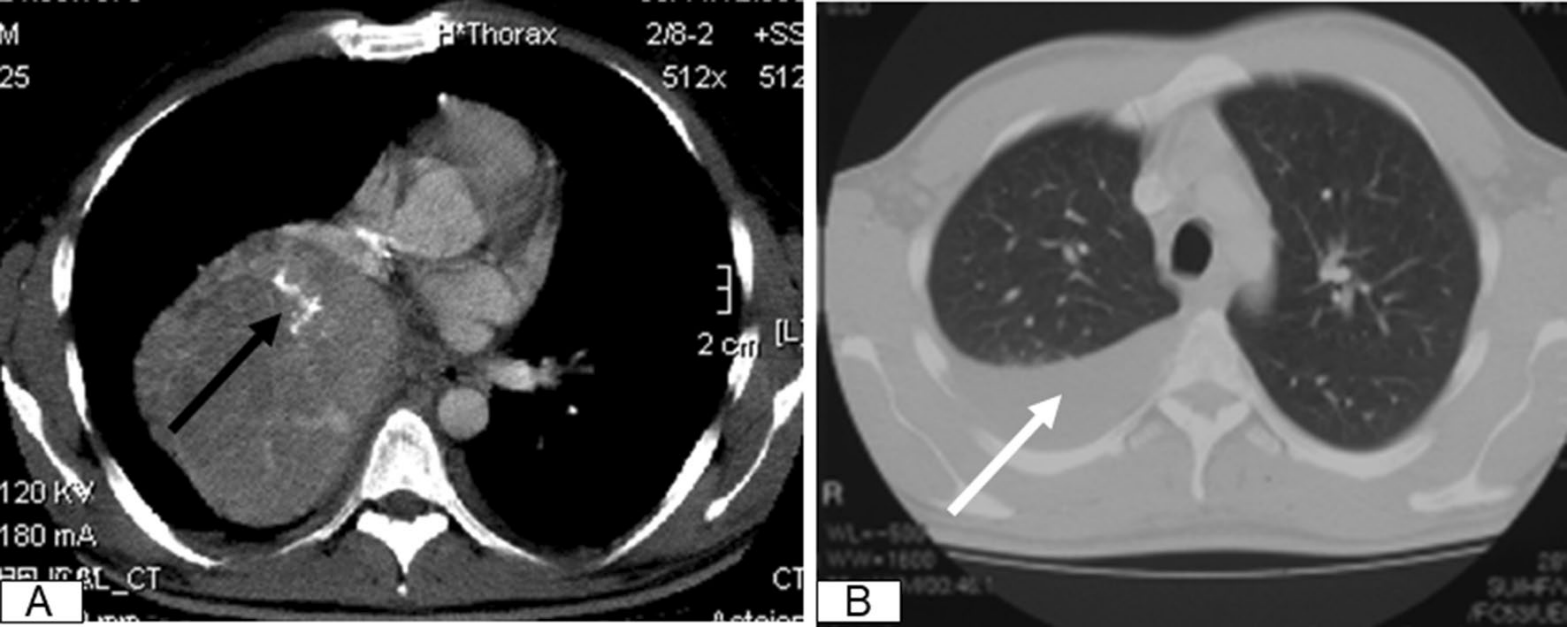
CT scan of the chest, showing the large tumor on the right side with calcification inside (black arrow) (a), accompanied pleural effusion (white arrow) (b)
